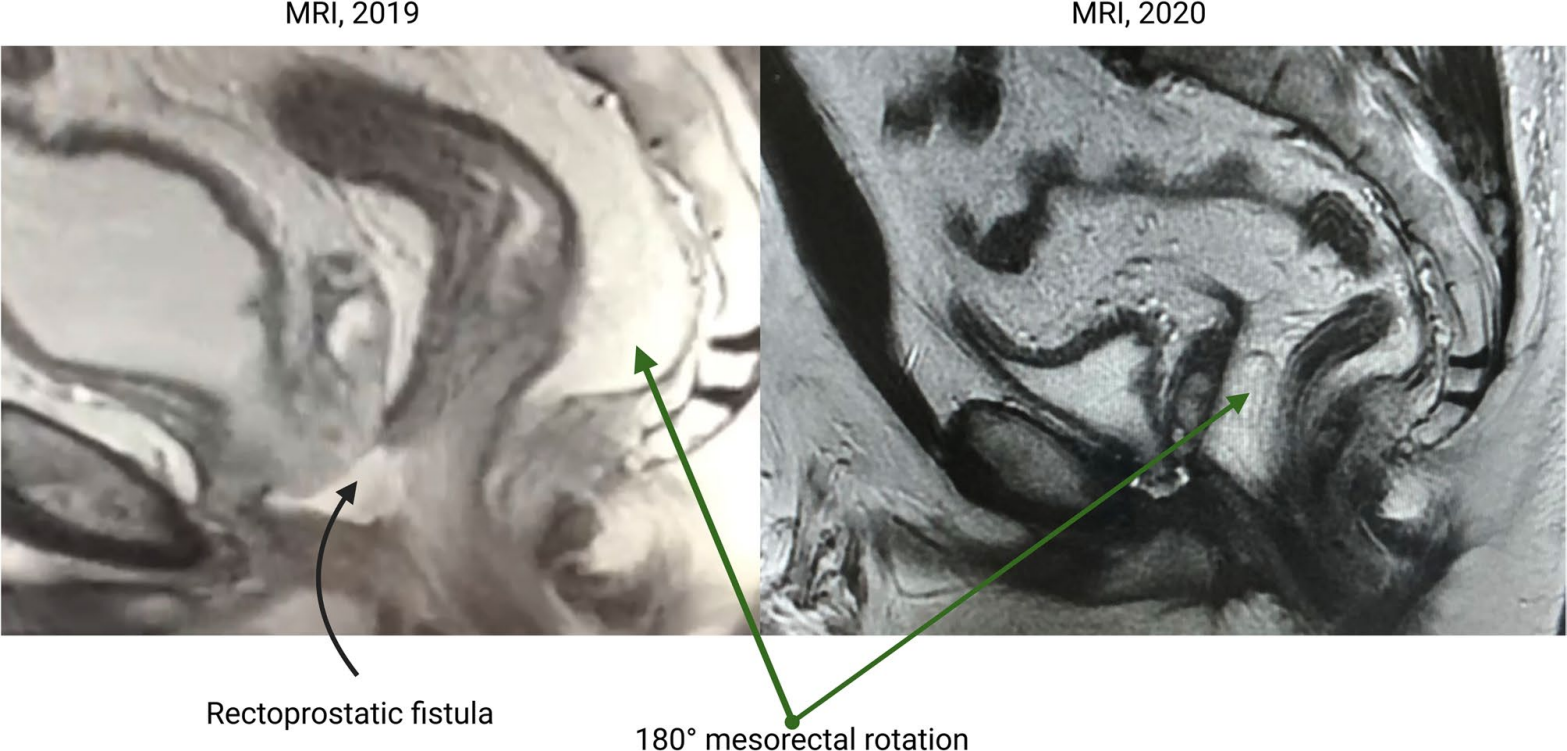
Comparative MRI scans from 2019 and 2020. The 2019 scan reveals a rectoprostatic fistula, while the 2020 scan demonstrates a 180° mesorectal rotation
